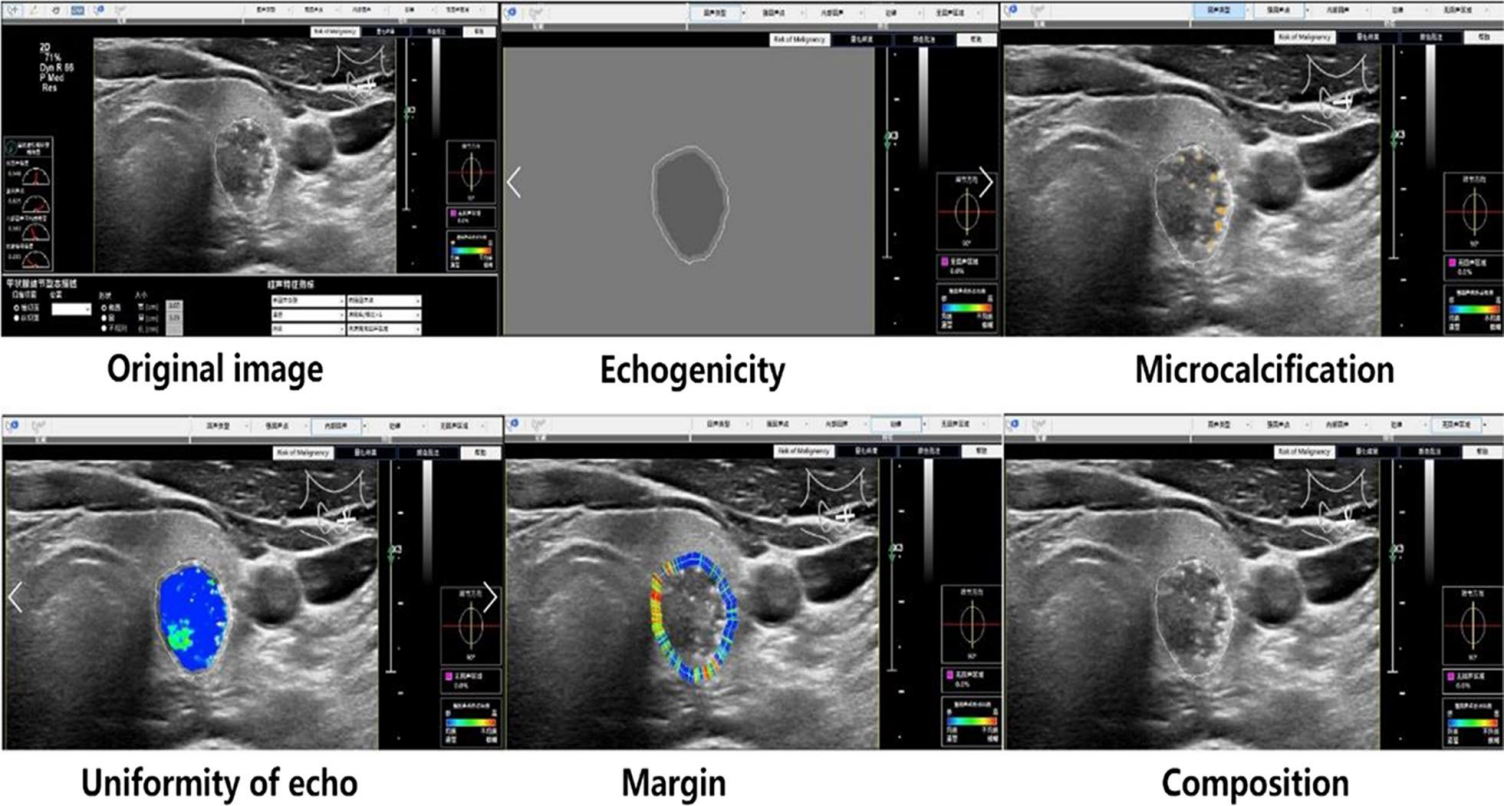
Automatic identification and quantization of ultrasound features of malignant thyroid nodules by the Am CAD-UT Detection System
